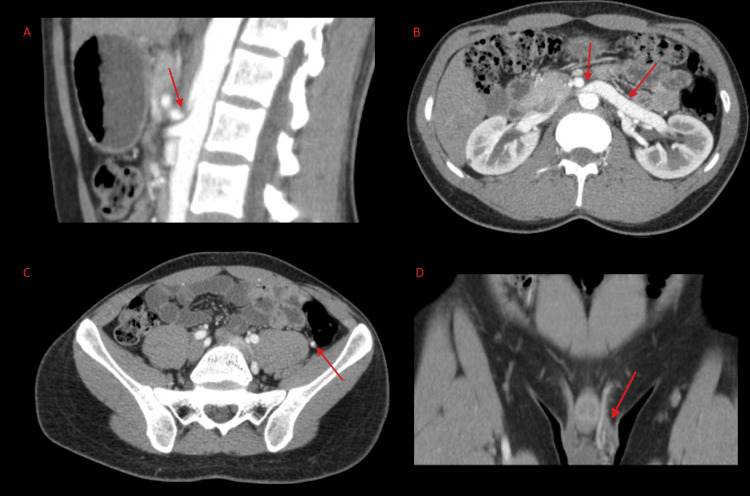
CT chest/abdomen with contrast (A) Sagittal CT abdomen with contrast demonstrating the median arcuate ligament compressing the celiac artery with apparent post-stenotic dilatation; arrow points to the stenosis point of the celiac artery. (B) Axial CT abdomen with contrast demonstrating a dilated SMA compressing left renal vein drainage, consistent with nutcracker syndrome; arrows point to the stenosis point of the left renal vein and dilatation of the left renal vein. (C) Axial CT abdomen with contrast demonstrating a dilated left spermatic vein compared to the right; arrow points to the dilated left spermatic vein. (D) Coronal CT abdomen with contrast demonstrating dilated pampiniform plexus veins, indicating a varicocele; arrow points to the dilated pampiniform plexus veins.
